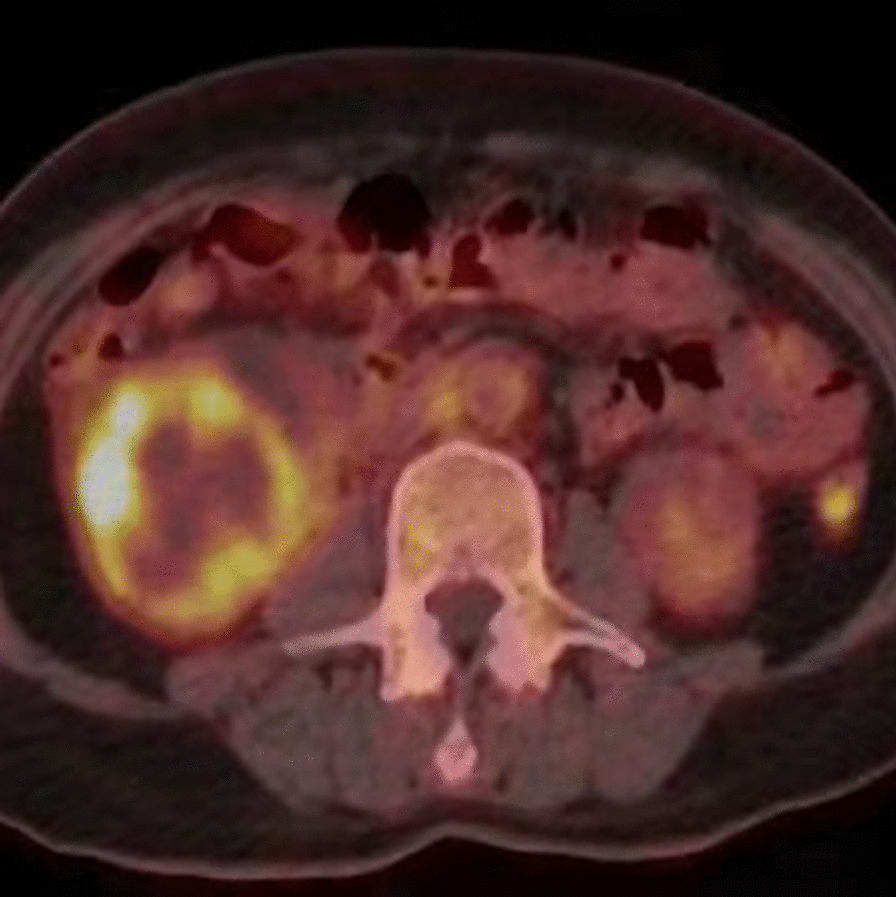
Axial ^18^F FDG PET/CT image of the lower abdomen
